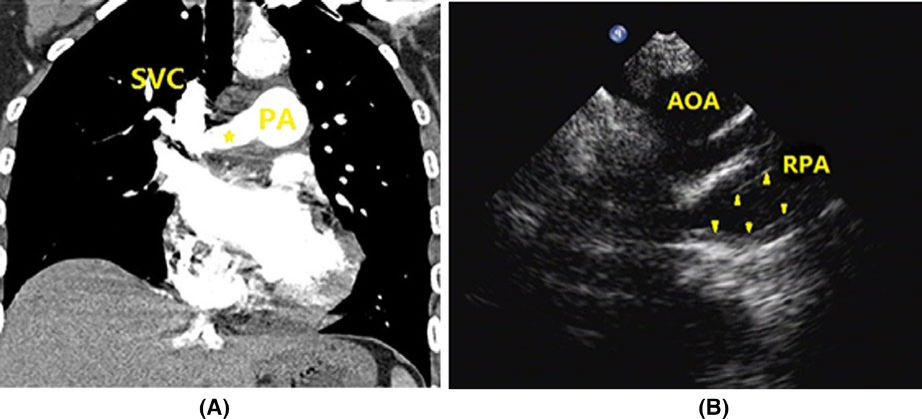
A. Coronal view by CTPA showed right pulmonary artery narrowing (asterisk *). B. Suprasternal right pulmonary artery long‐axis view showed right pulmonary artery extensive intimal thickening and luminal stenosis (arrowheads). SVC = superior vena cava; RPA = right pulmonary artery; AOA = aortic arch; CTPA = computed tomographic pulmonary angiography
